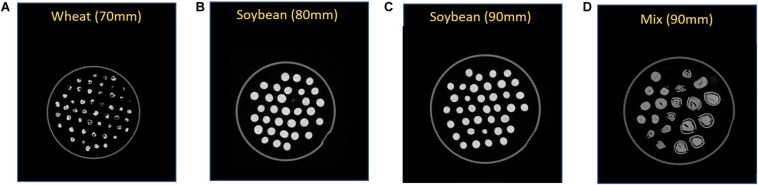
The raw X-ray computed tomography images. (A) wheat seeds in the container with a diameter of 70 mm; (B) soybean seeds in the container with a diameter of 80 mm; (C) soybean seeds in the container with a diameter of 90 mm; (D) peanuts, pine nuts, and pistachio nuts in the container with a diameter of 90 mm.
